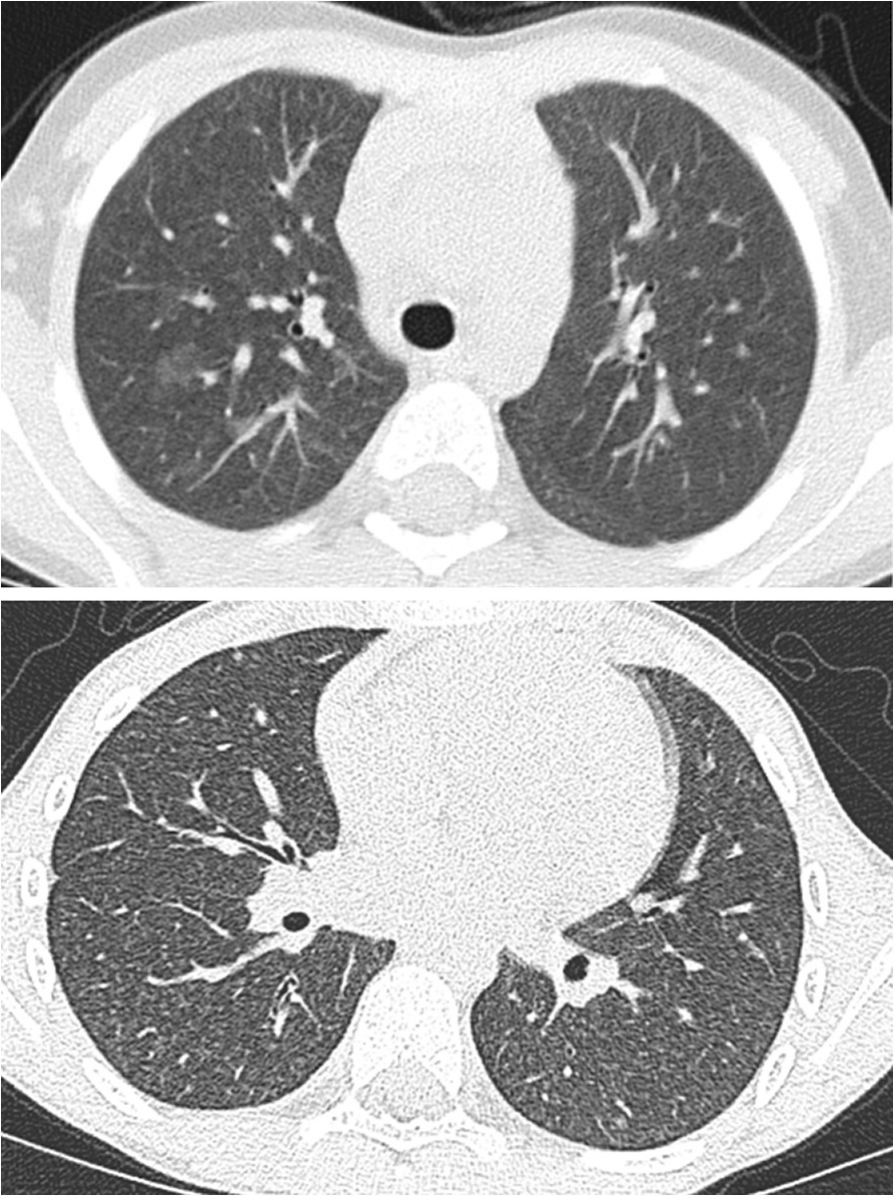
HRCT findings of HP. Upper panel shows acute HP with ground-glass opacification more prominent on the right side, the lower panel shows diffuse micronodules and ground-glass attenuation in subacute HP.
